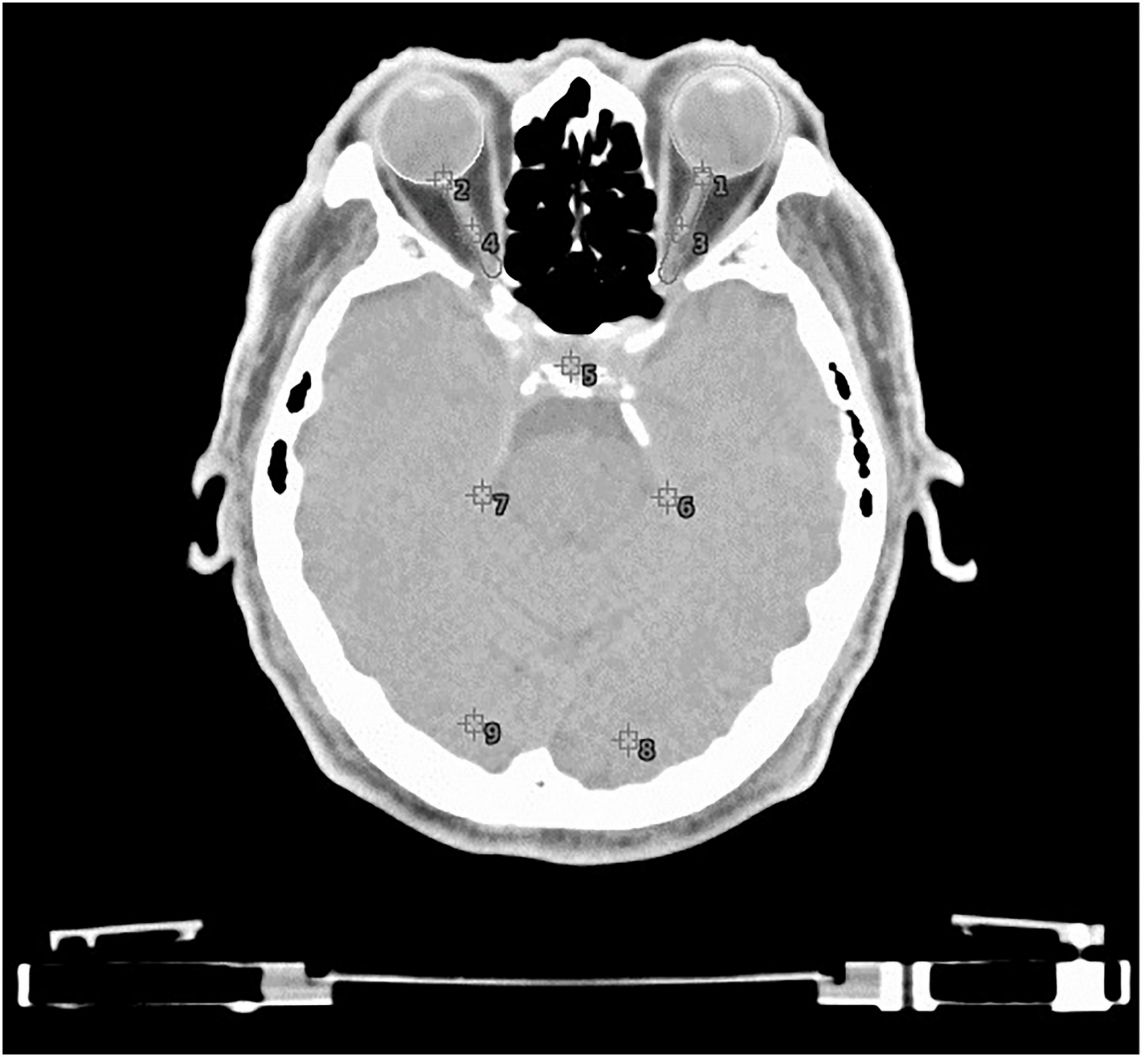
The point-dose method to analyze the fraction dose of the computed tomography axial view.
